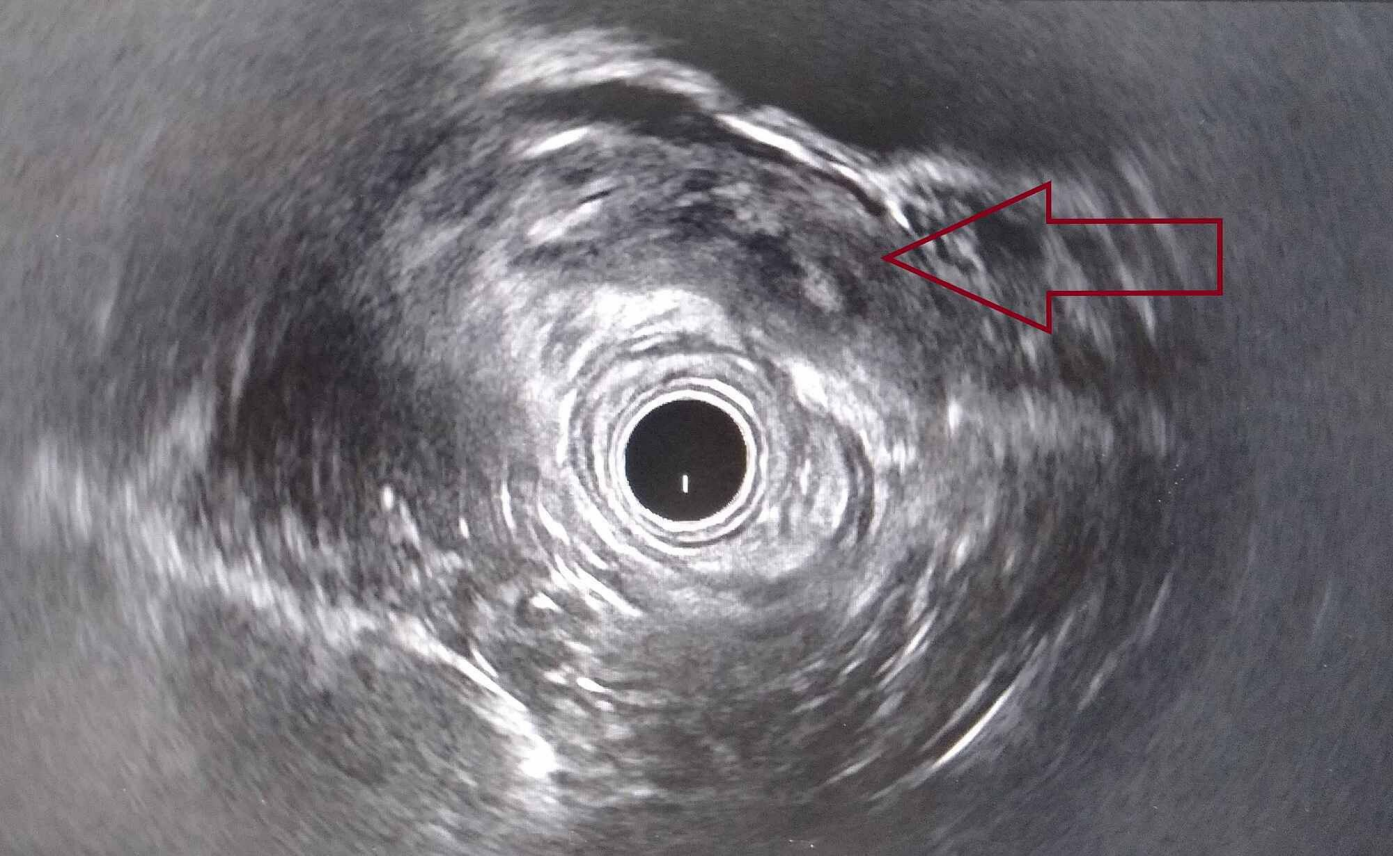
Rectal endoscopic ultrasound showing a well-limited, hypoechoic, and heterogeneous mass of the right lateral rectal wall (red arrow)
